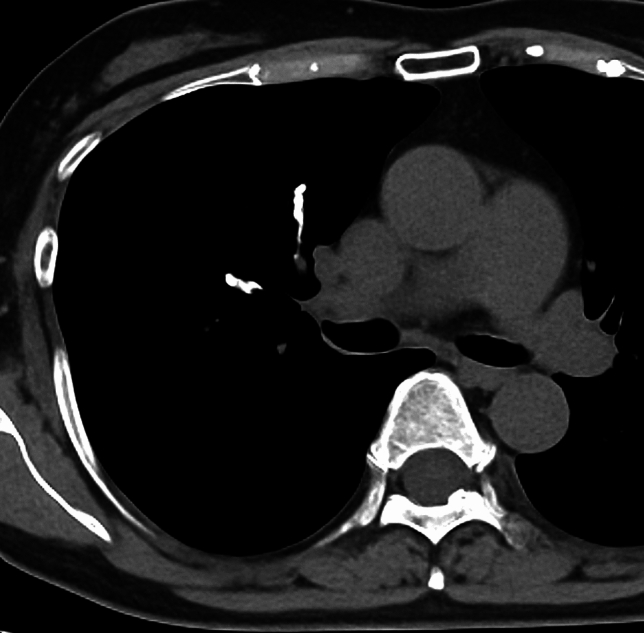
N-butyl-2-cyanoacrylate (NCBA) pulmonary embolism. A woman in her 60 s underwent the embolization of a renal arteriovenous fistula with the use of NBCA-Lipiodol®. During the embolization procedure, embolic material migrated from the dilated renal vein into the inferior vena cava. Chest CT subsequently revealed a hyperdense foreign body in the right pulmonary artery, consistent with a pulmonary embolism caused by NBCA-Lipiodol migration
